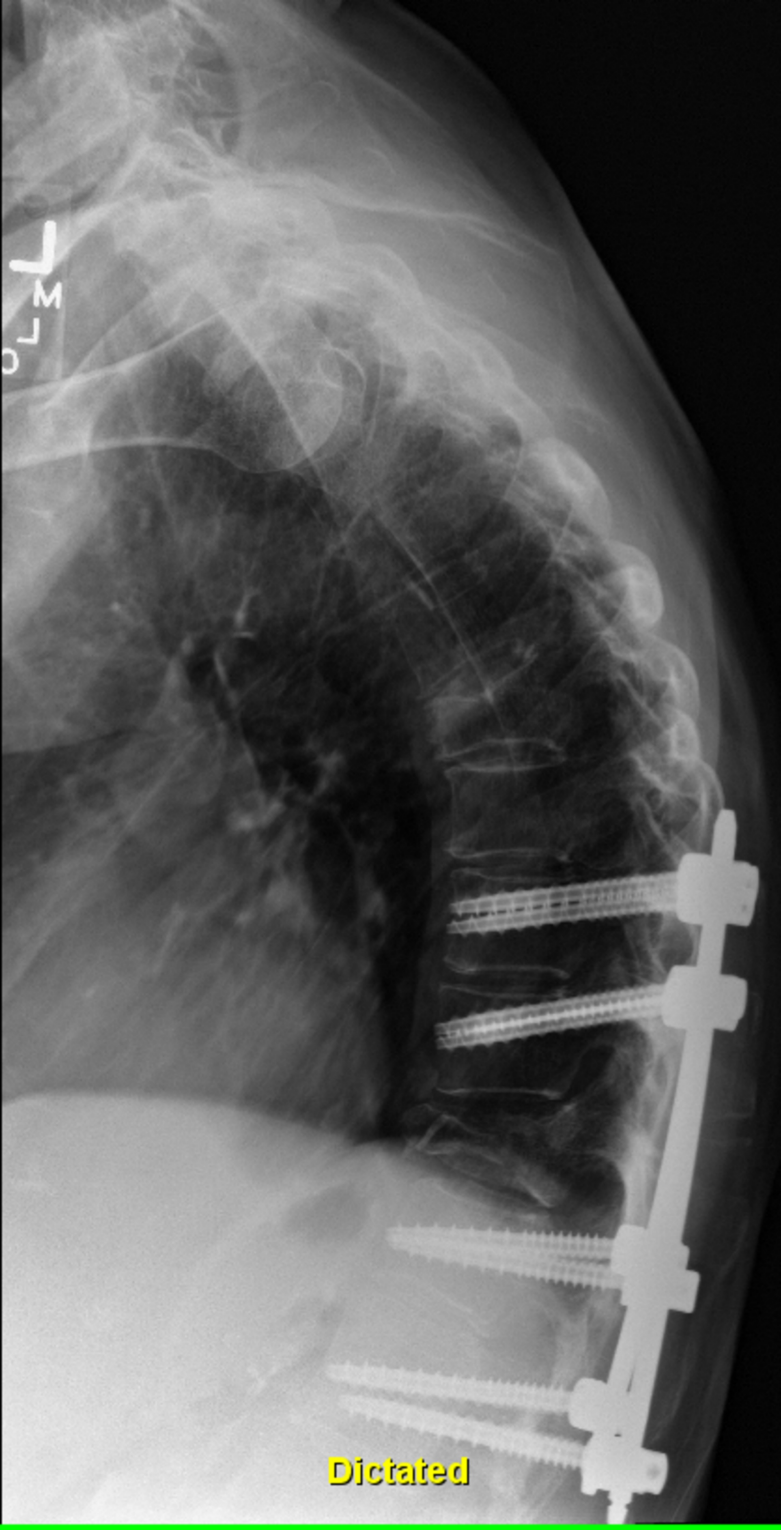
Three months post-operative thoracic spine X-ray with intact hardware without lucency
